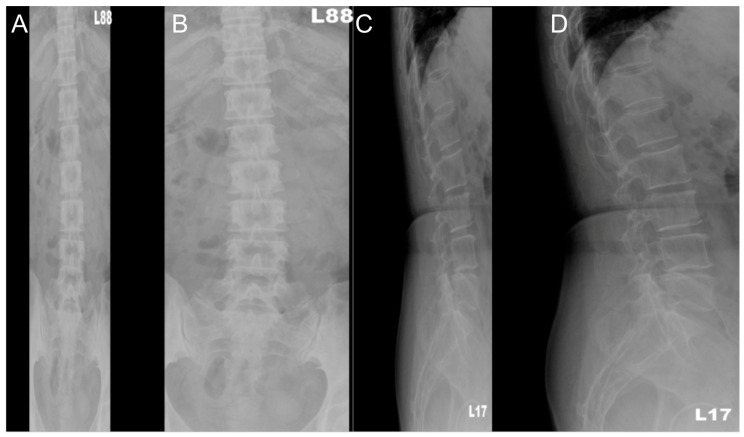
Effect of image cropping on AP and LAT plain radiographs. (A,C) Original images before cropping. (B,D) The same images after proper cropping, which removes irrelevant background while preserving all critical anatomic structures. Care must be taken to avoid over-cropping, which can remove diagnostically important regions.
